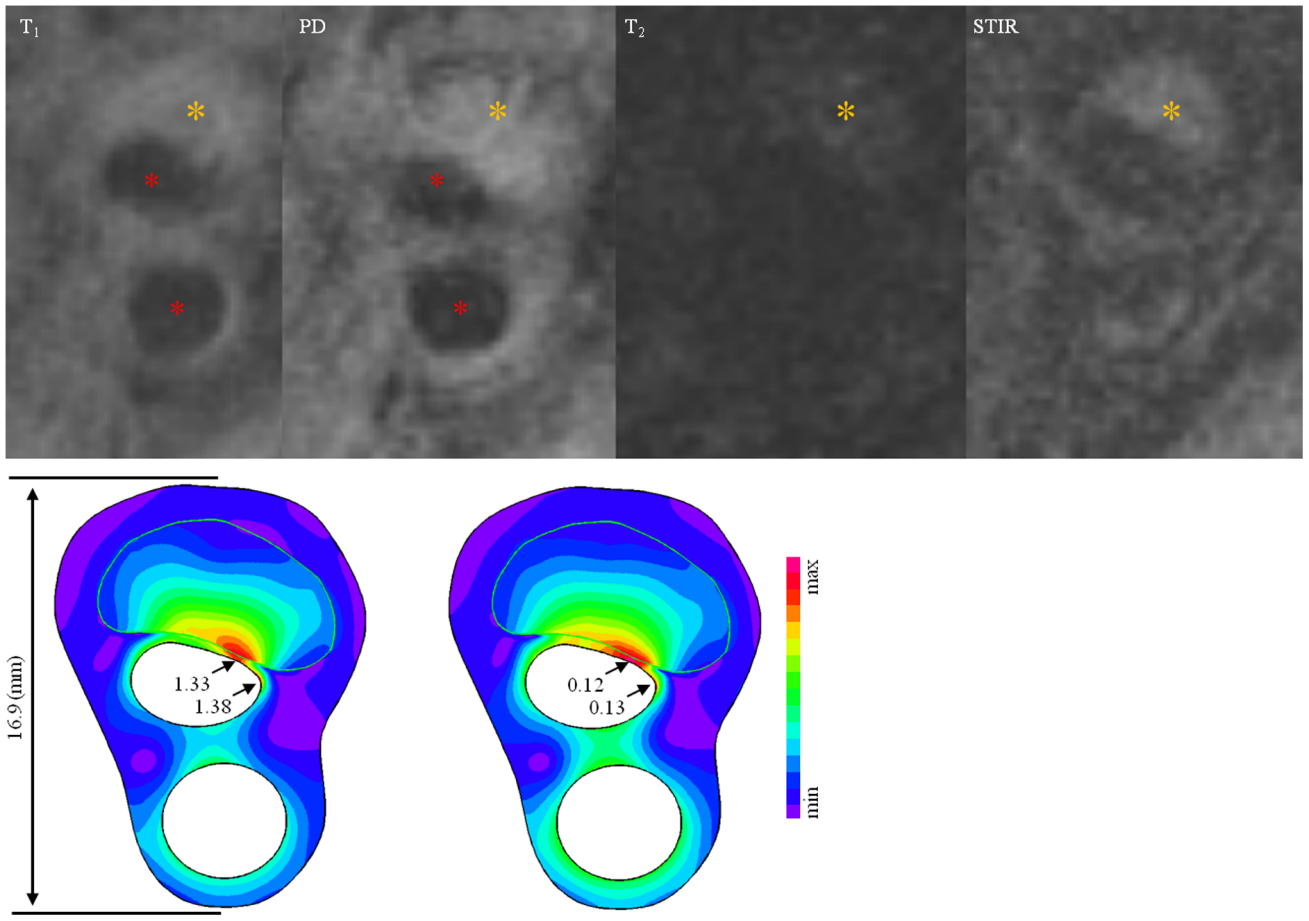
In vivo MR images and band plot of computational results [The 1^st^ row: Signal intensity of a plaque near the carotid bifurcation under T_1_, PD, T_2_ and STIR MR sequences (red asterisks stand for lumen and the brown for fresh intraplaque haemorrhage); the 2^nd^ row, the left image is the band plot of Stretch-P_1_ at systole and the right one is the variation of Stretch-P_1_ during one cardiac cycle].
